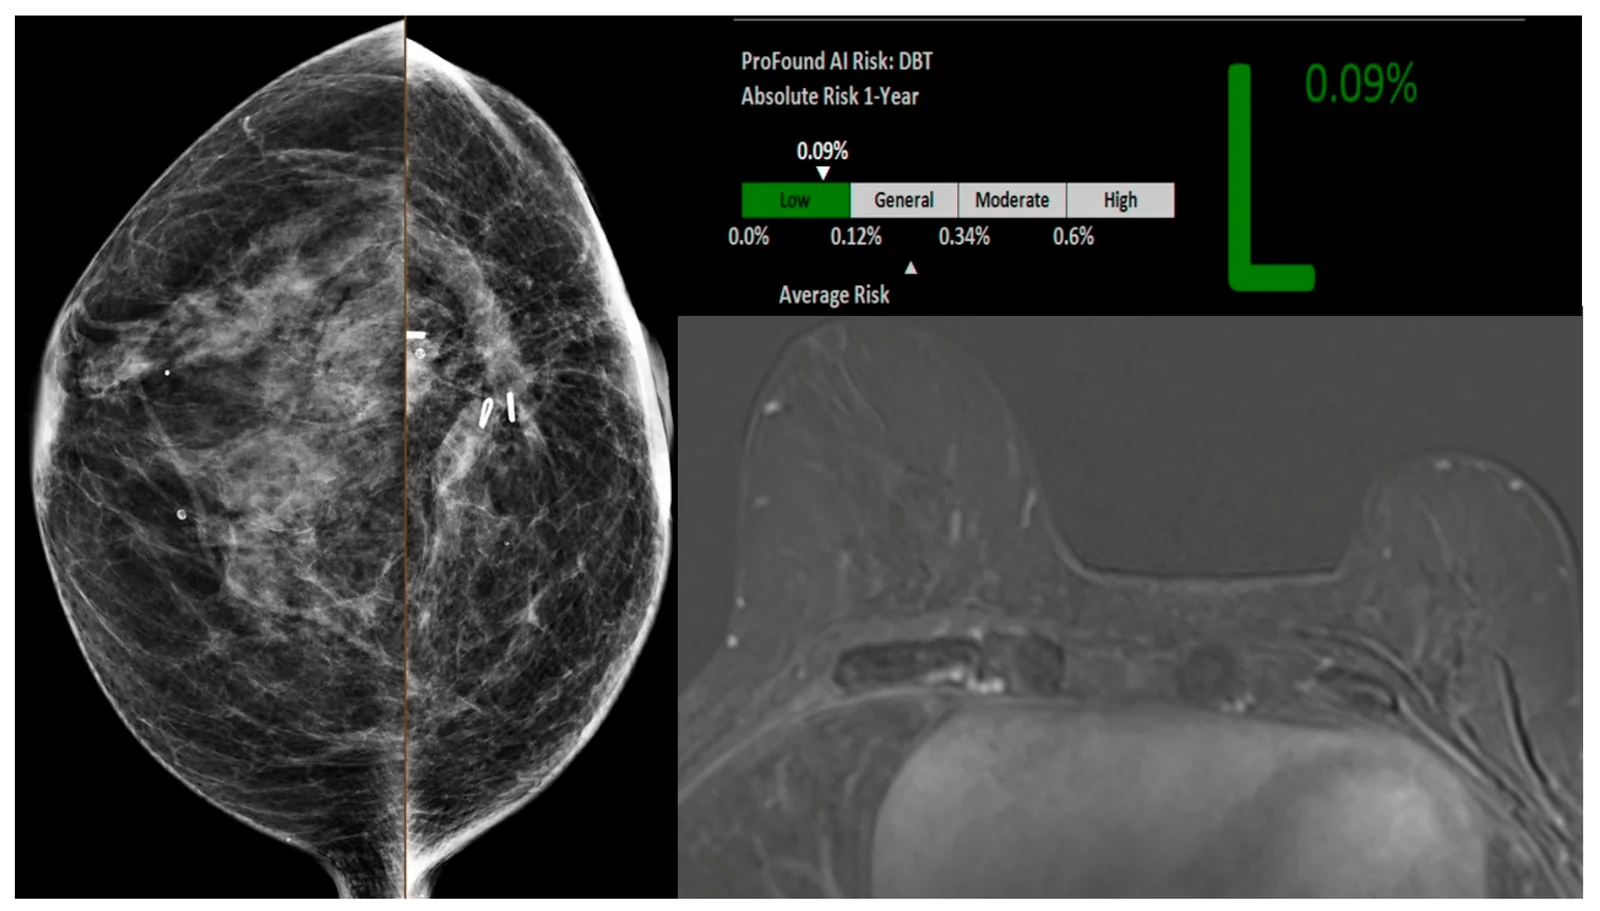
(No subsequent breast cancer/Control): 63-year-old with a history of left breast HER2-positive IDC 3 years prior, status post lumpectomy, adjuvant chemoradiation, and endocrine therapy. CC images from the index screening mammogram show heterogeneously dense tissue and lumpectomy changes in the left breast, assessed as BI-RADS 2. Patient underwent high-risk screening breast MRI, which was benign (BI-RADS 2). The AI risk score from the screening mammogram was low.
